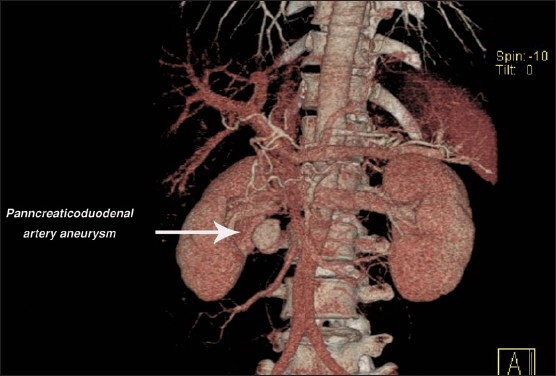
CT angiography slowing the pancreaticoduodenal artery aneurysm.
